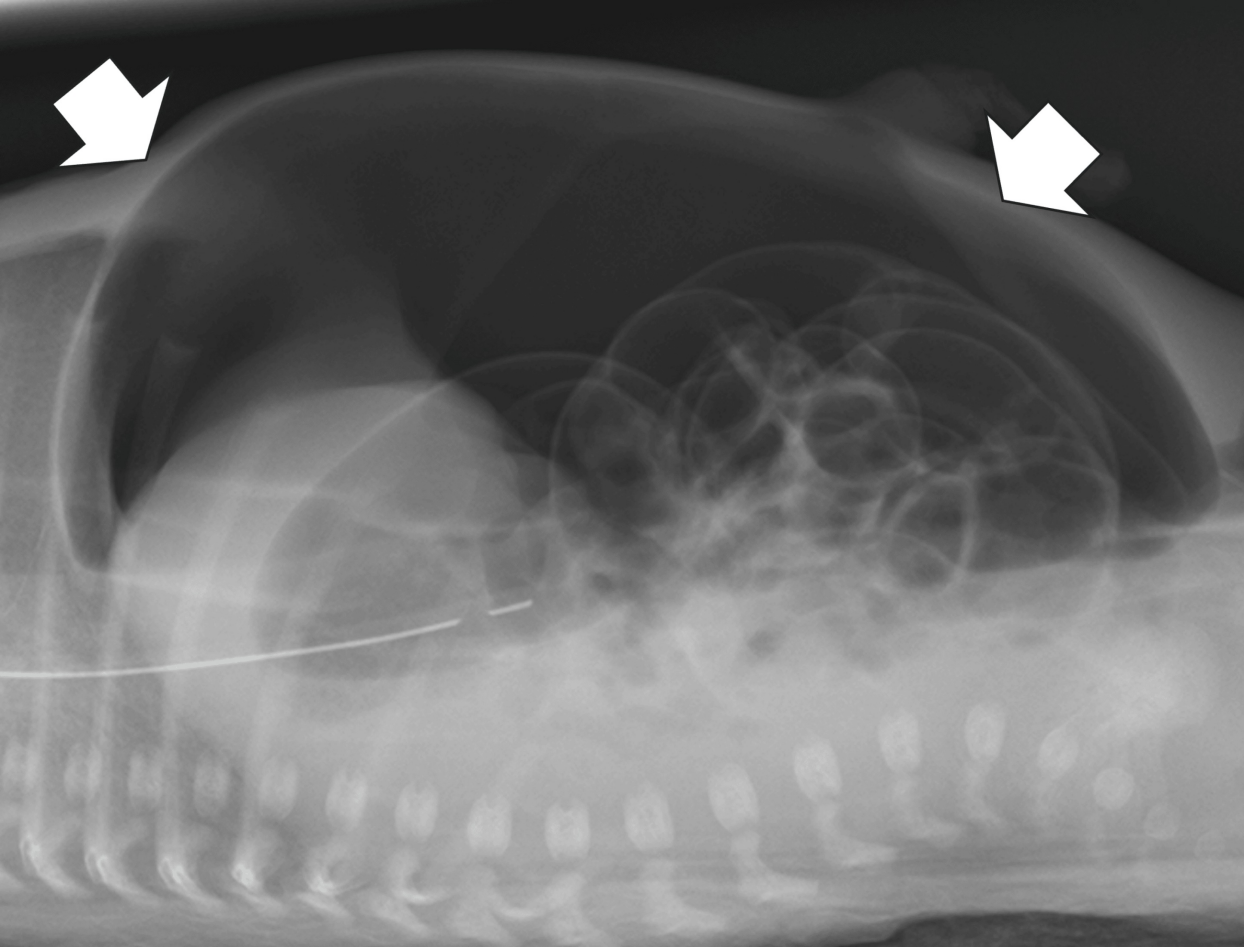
Abdominal radiograph finding in the cross-table lateral view position on day of life eight. Figure shows free air on a supine cross-table lateral view (white arrow).
